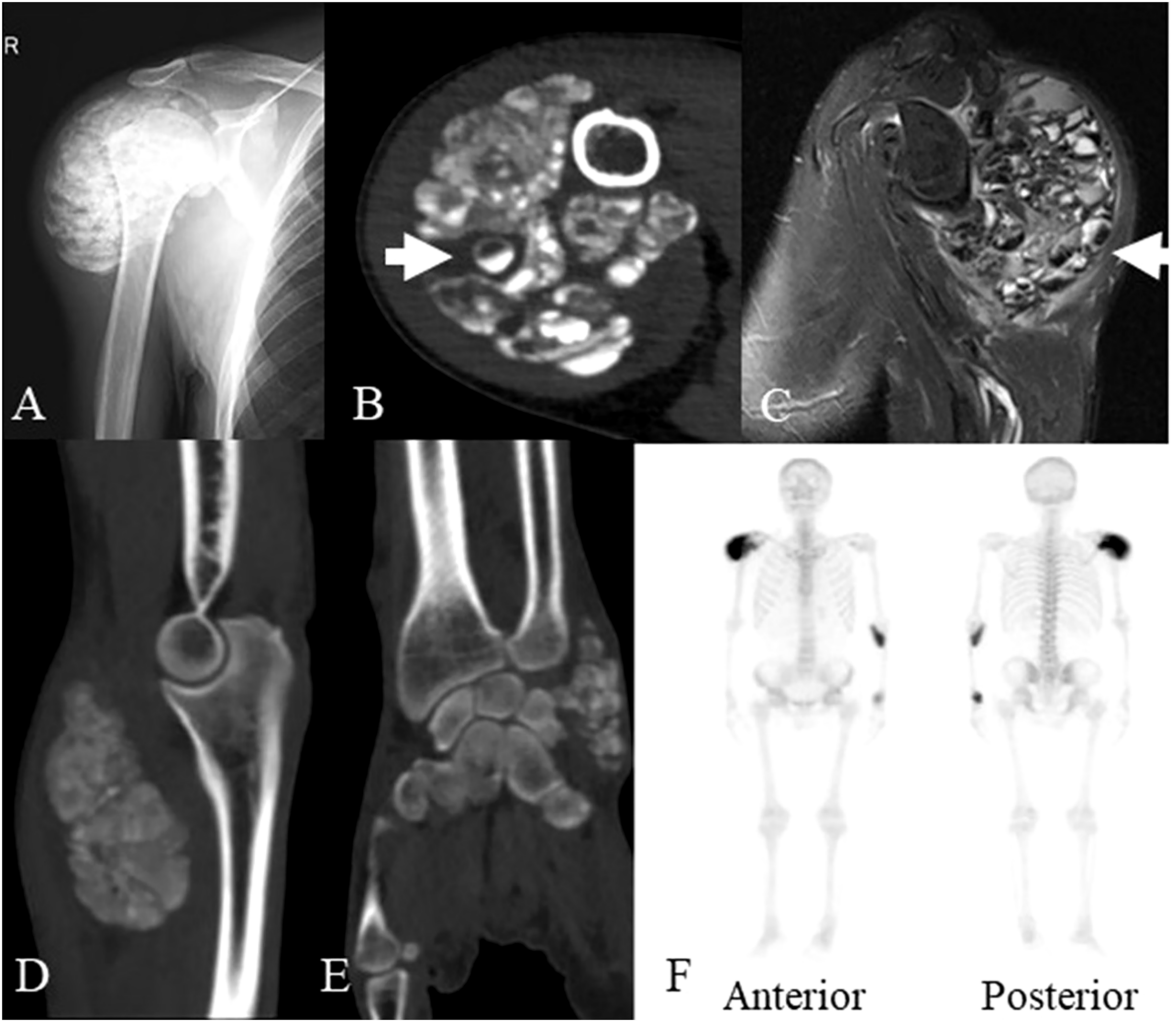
The imaging data of the patient at the age of 45 years old. (A) X-ray showed a lobulated calcified mass in the soft tissues around the right shoulder joint. (B) Axial CT scan showed a lobulated partially calcified mass around the right shoulder joint, some of which demonstrated cysts with sedimentation sign (arrow). (C) MRI showed mixed signal mass in soft tissues around right shoulder joint, some of which also demonstrated cysts with sedimentation sign (arrow). (D) Sagittal planes CT scan showed a lobulated calcified mass around the left elbow joint. (E) coronal planes CT scan showed a lobulated calcified mass around the left wrist joint. (F) SPECT revealed the increased accumulation of radiotracer in the masses of the right shoulder, left elbow and wrist. CT, computed tomography; MRI, magnetic resonance imaging; SPECT, single photon emission computed tomography.
